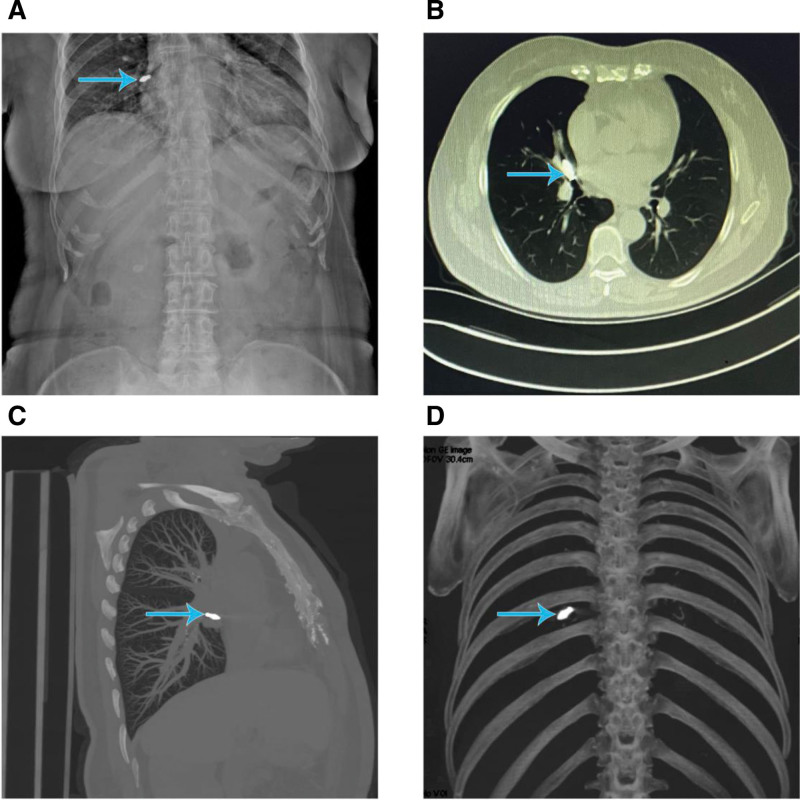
Airway foreign body in a 69-yr-old female patient. The blue arrow indicates the foreign body within the bronchus. (A) Upright abdominal radiograph showing a high-density shadow in the right hypochondriac region. (B–D) Chest CT scan with 3-dimensional reconstruction demonstrating impaction of the crown–abutment assembly at the bifurcation of the right middle lobe bronchus. CT =  computed tomography.
